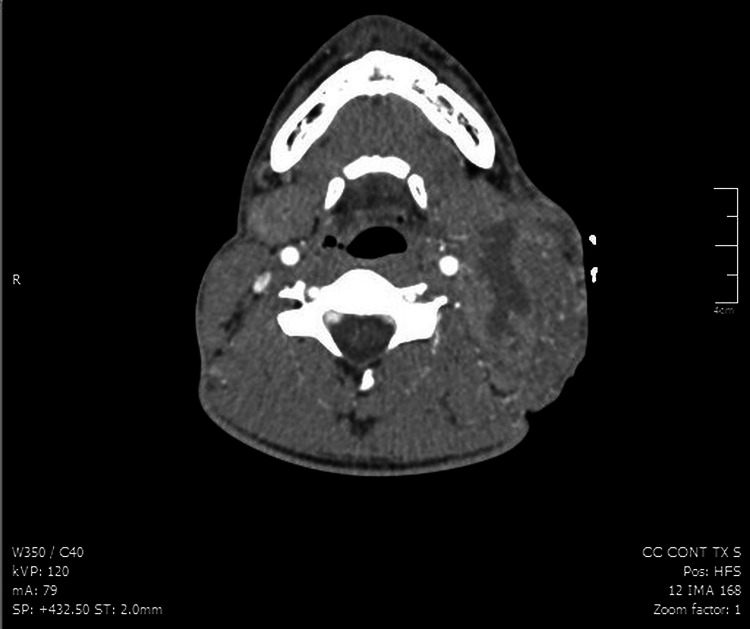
Neck CT showing a left lymph node conglomerate with hypodense images due to necrotic changes, loss of cleavage plane with muscle structures, and mass effect on the ipsilateral jugular vein. CT: computed tomography
